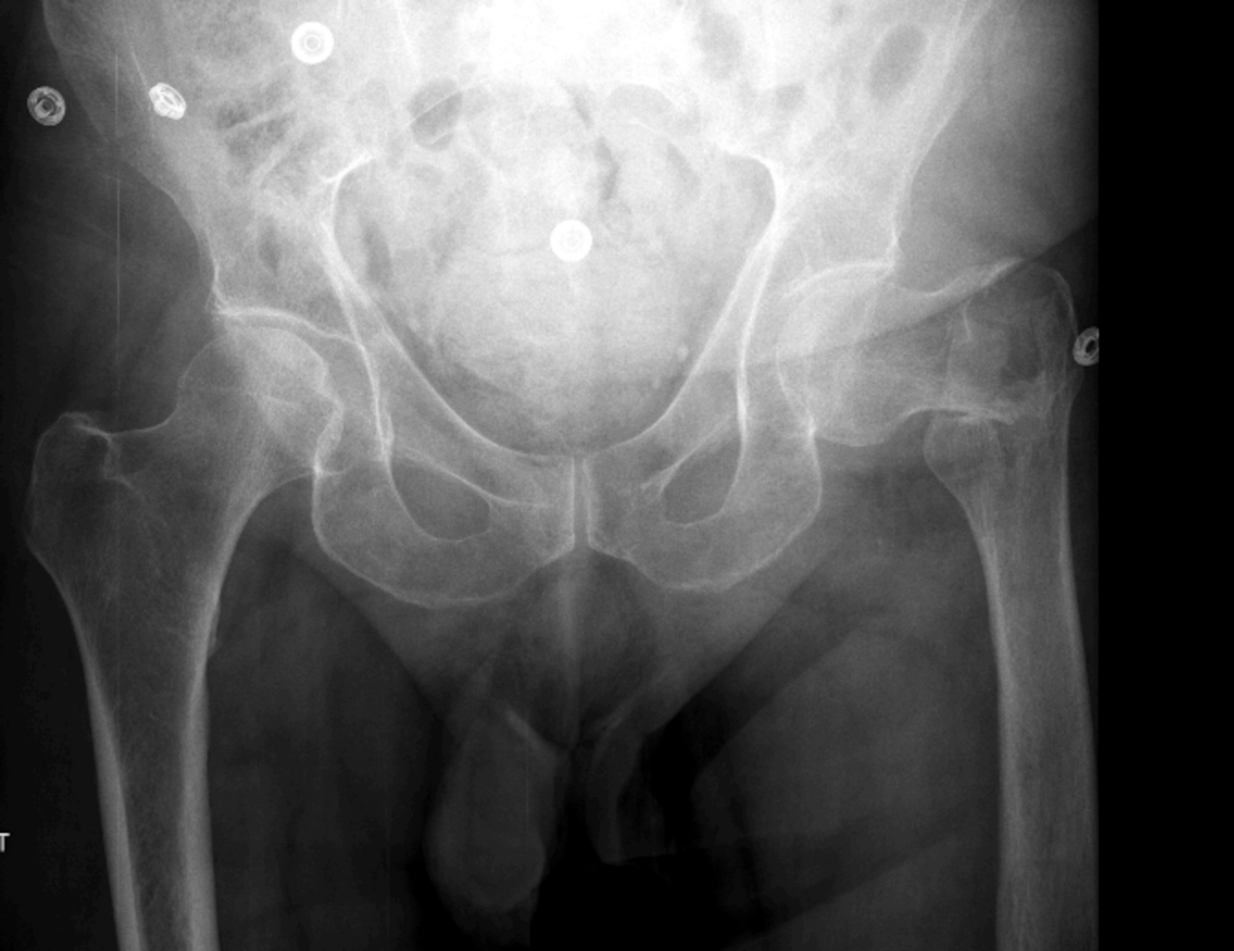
Pelvic X-ray anteroposterior view The image shows the left extracapsular intertrochanteric neck of femur fracture
